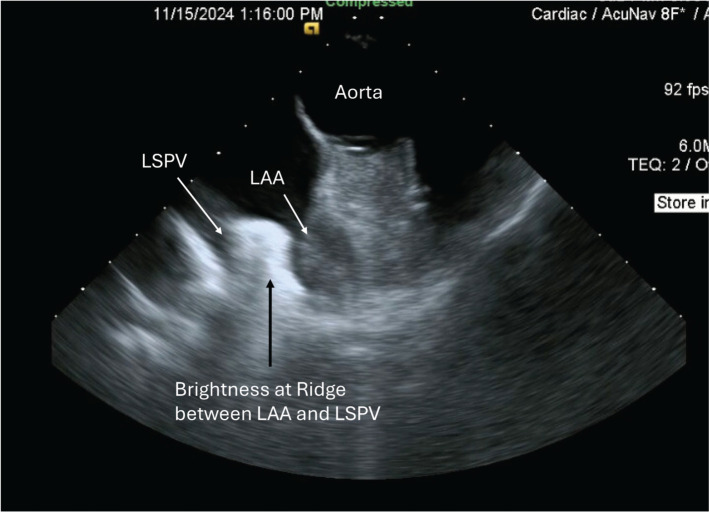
Intracardiac echocardiogram appearance after ethanol injection. Intracardiac echocardiogram short-axis view from the right ventricular outflow tract reveals the brightness at the ridge between the left atrial appendage and left superior pulmonary vein. *Abbreviations:* LAA, left atrial appendage; LSPV, left superior pulmonary vein.
